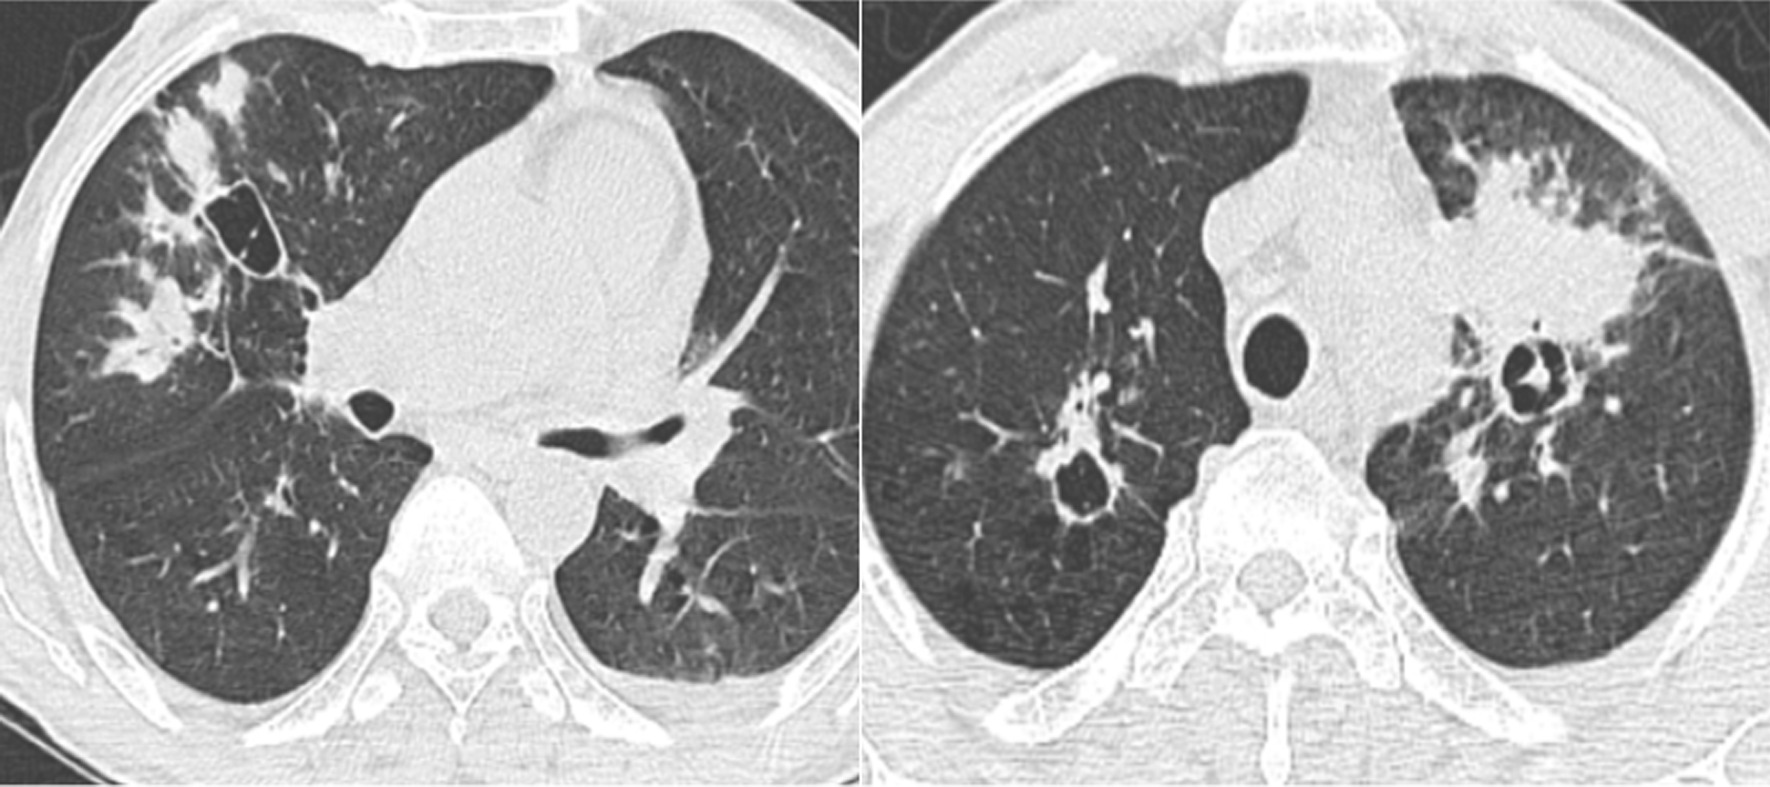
Thoracic CT scan, parenchymal window, axial sections: multiple excavated intraparenchymal lung nodules, irregularly contoured, thick-walled
